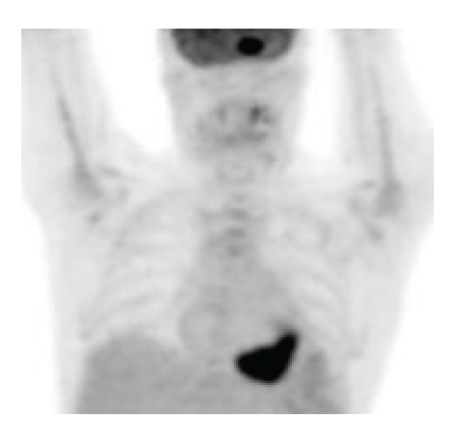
PET-CT demonstrated a focus of avidity at the known cerebellopontine lesion, as well as in the posterior inferior wall and papillary muscle of the left ventricle of the heart.
